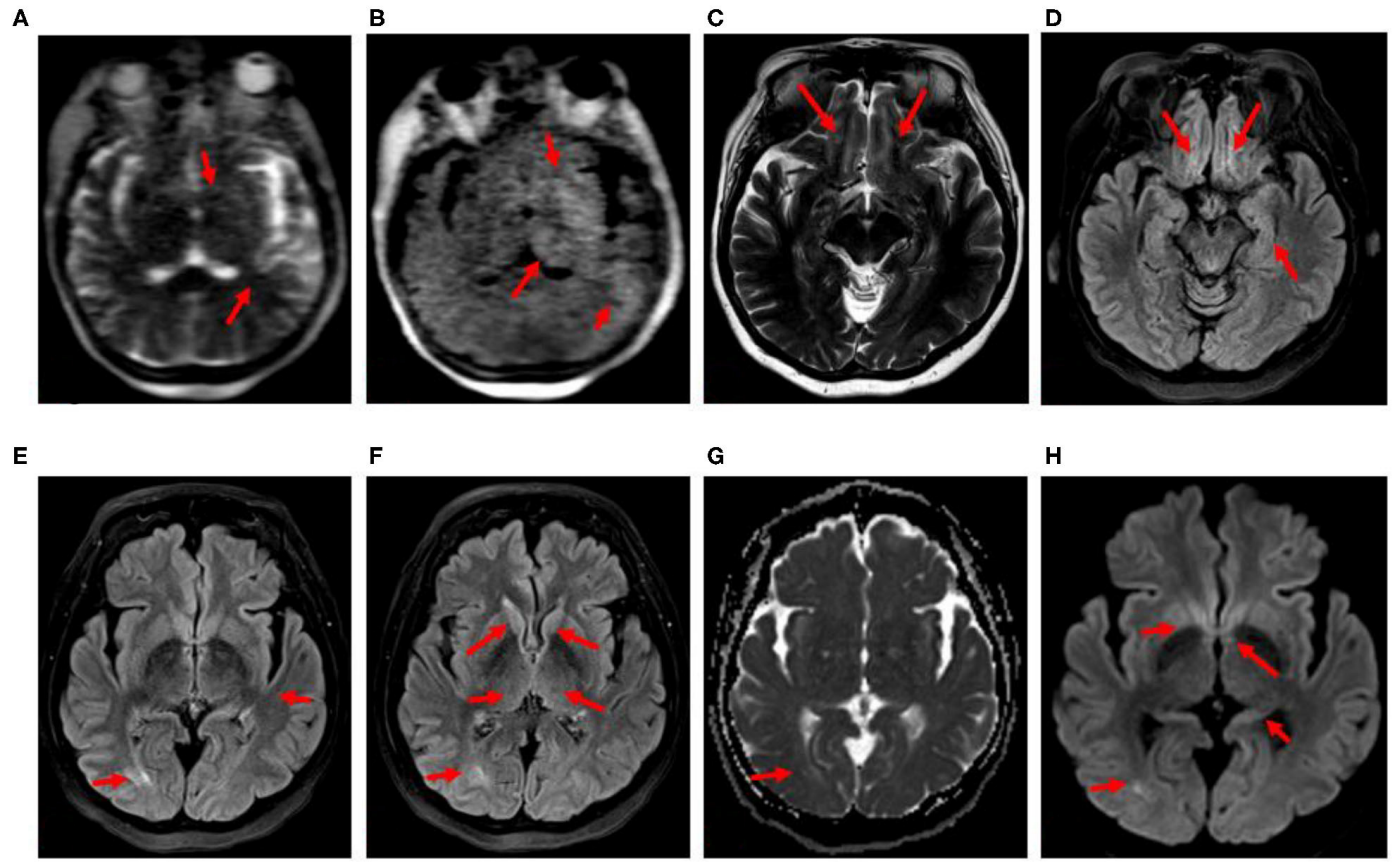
(A–H) Case 4: 68-year-old, COVID-positive female. (A,B) Hyperfine 0.064-T portable MRI obtained 26 days after admission at the patient's bedside showing focal T2 and FLAIR hyperintensities (red arrows). (C–H) 3-T MRI obtained 39 days after admission. (C) Axial T2 and (D) axial FLAIR hyperintensities (red arrows): left subinsular cortex, lentiform nuclei, thalami, and left temporal lobe. (E,F) Axial FLAIR, (G) axial ADC, (H) axial DWI, T2, FLAIR, and DWI hyperintensities (red arrows): bilateral olfactory tracts, medial temporal lobes including hippocampal tails, caudate heads, fornices and posterior commissural fibers, right posterior lateral temporal lobe without restricted diffusion.
